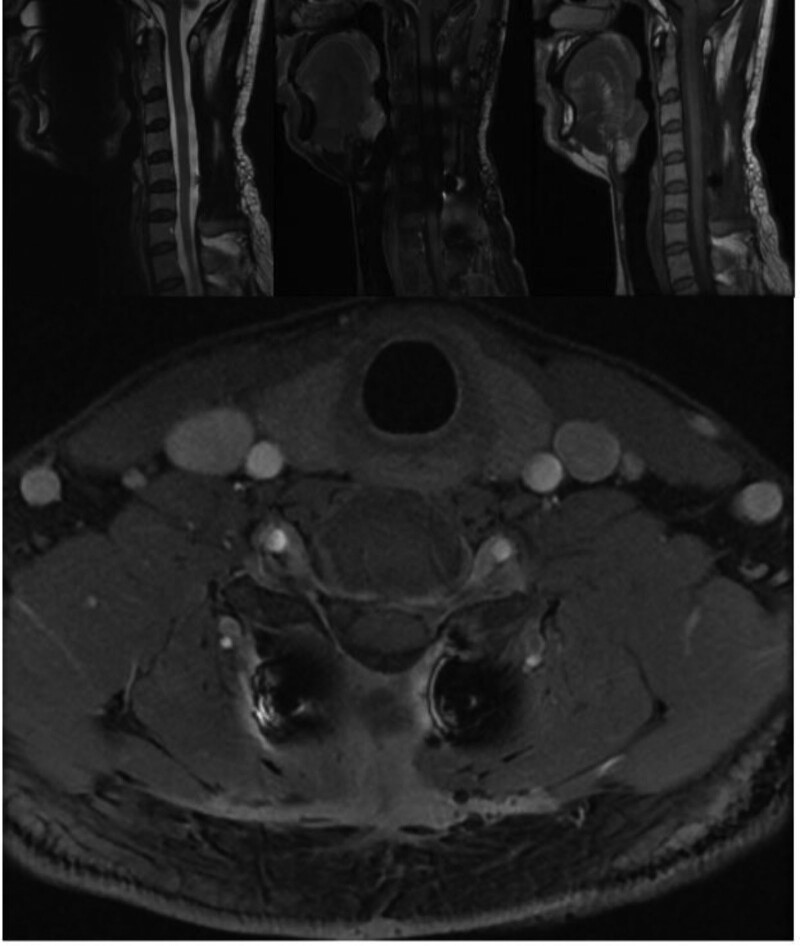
Third postoperative magnetic resonance imaging 10 mo after surgery.
